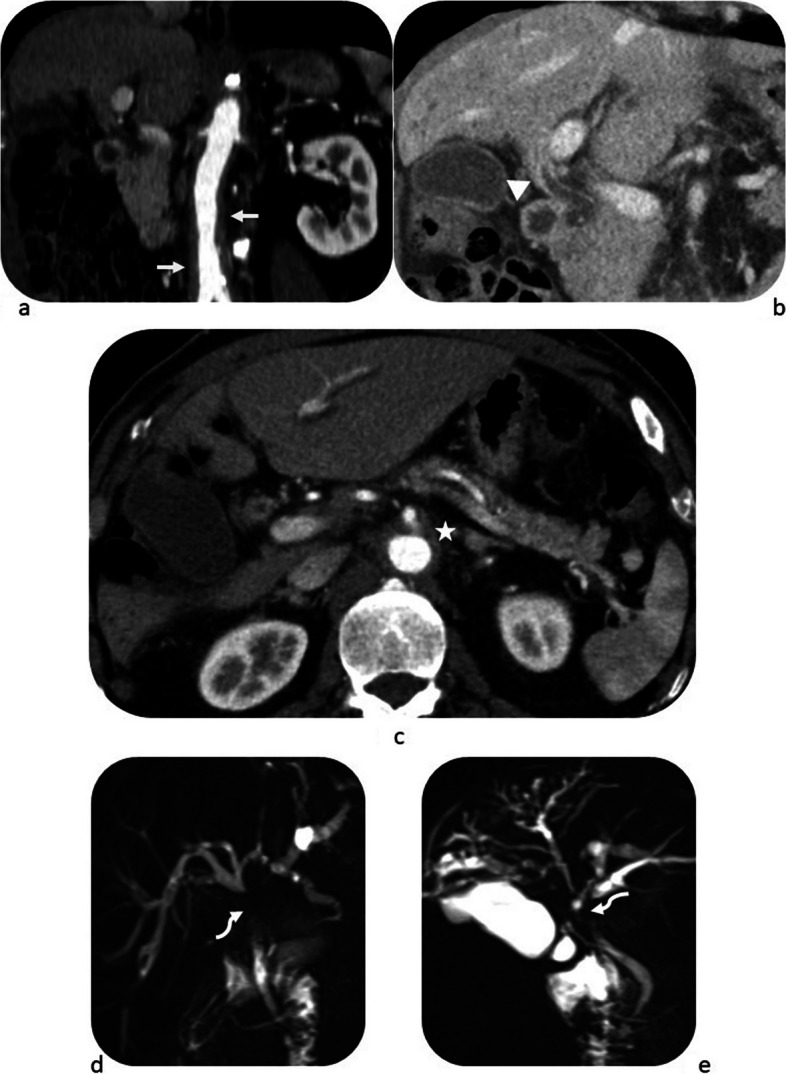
Case of pancreaticobiliary involvement scoring 19, periaortitis scoring 4 points and elevated serum IgG_4_ scoring 4 points. Overall score = 27 points. a Coronal reformat arterial phase CT image shows symmetrical wall thickening of the aorta involving the infrarenal aorta (white arrows). b Coronal reformat portal venous CT image shows mural thickening and hyperenhancement of the CBD wall (white arrowhead). c Axial arterial phase CT image shows concentric wall thickening of the aorta in keeping with periaortitis (white star). d, e Two views of coronal MRCP show a stricture involving the CBD over a 3-cm segment (curved arrows), with upstream intrahepatic biliary duct dilatation
